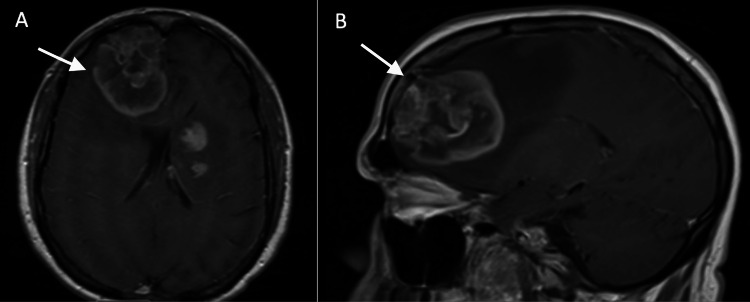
Contrast-enhanced magnetic resonance imaging of the lesion A) Axial T1 weighted image; B) Sagittal T1 weighted image depicting a large, heterogeneously enhancing 6 x 4.3 x 5 cm right frontal lobe mass with subfalcine herniation and 1 cm midline shift to the left
